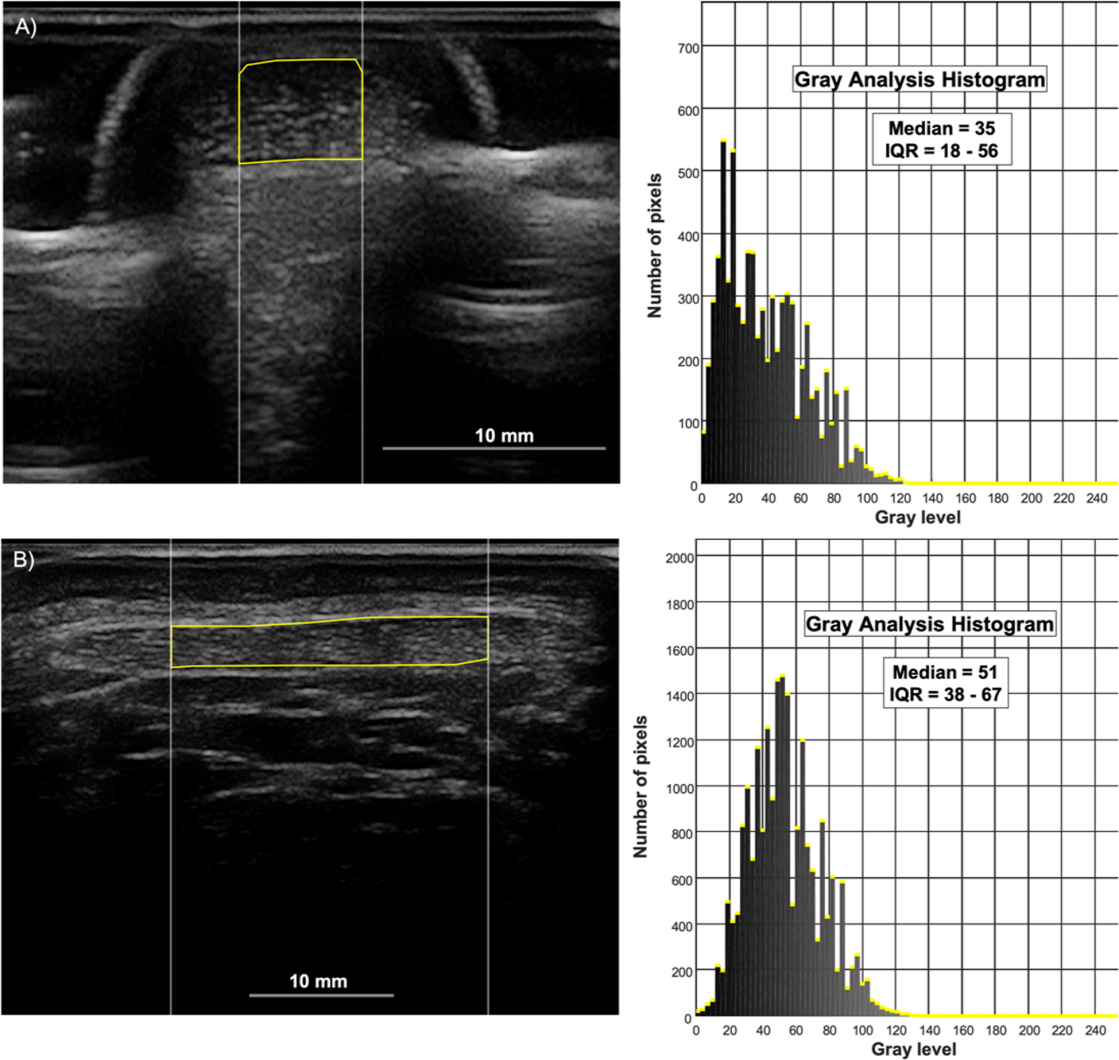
a) Transverse B-mode ultrasound image of the Achilles tendon with corresponding gray scale histogram. b) Transverse B-mode ultrasound image of the patellar tendon with corresponding gray scale histogram
